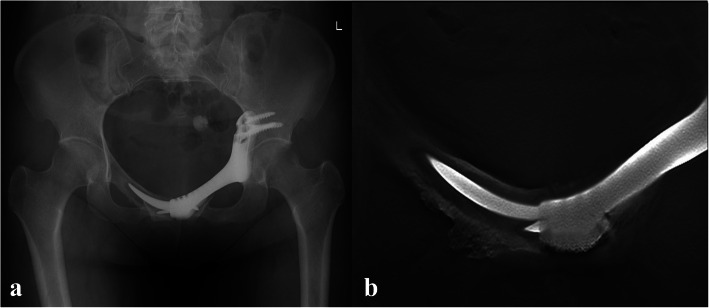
Six months after operation, X-ray (a) and T-SMART (b) showed fretting wear appeared around prosthetic stem
